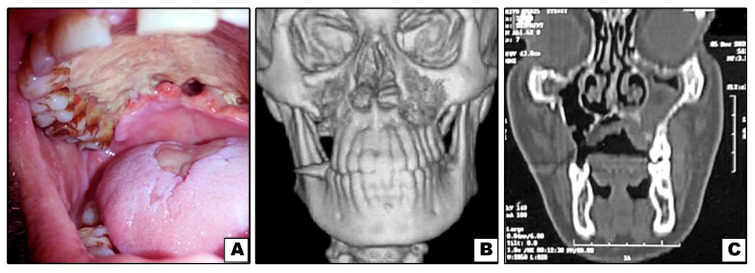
Case#3: (A) Exposed necrotic palatal bone with palatal perforations. (B,C) 3D and coronal CT showing bone destruction of right and left maxillae (not involving inferior orbital rims).
